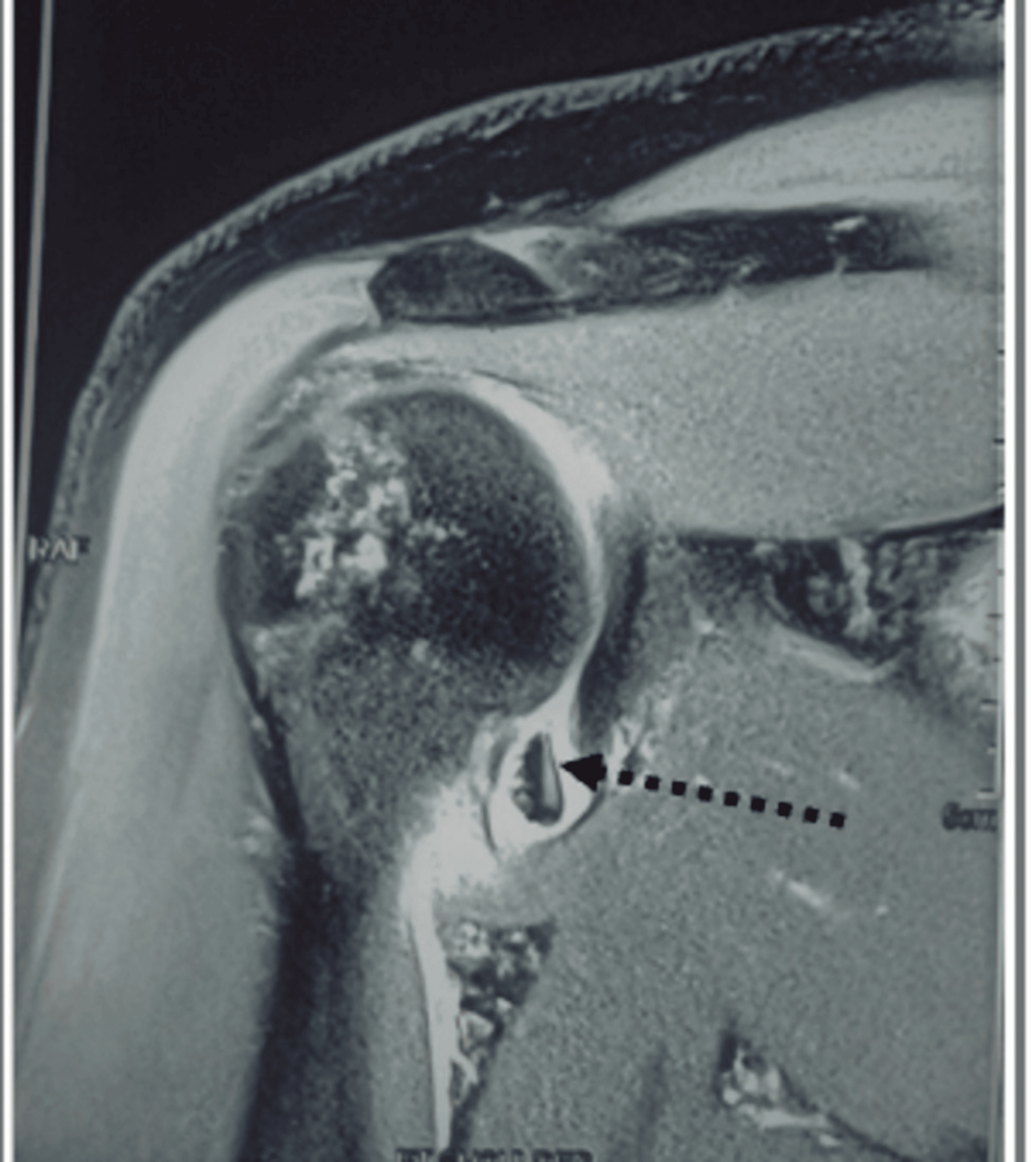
MRI coronal plane showing a free chondral fragment in the joint (black dotted arrow).
